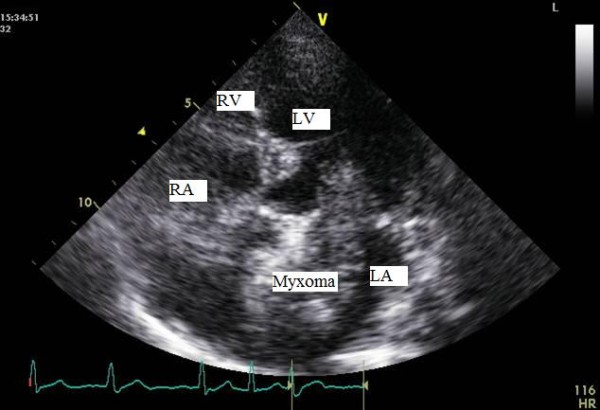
Transthoracic echocardiographic (TTE) image of the left atrial myxoma. The figure shows the tumor in the left atrium (LA) in the apical four chamber view, showing the four cardiac chambers (LA: left atrium, RA: right atrium, LV: left ventricle, RV: right ventricle).
